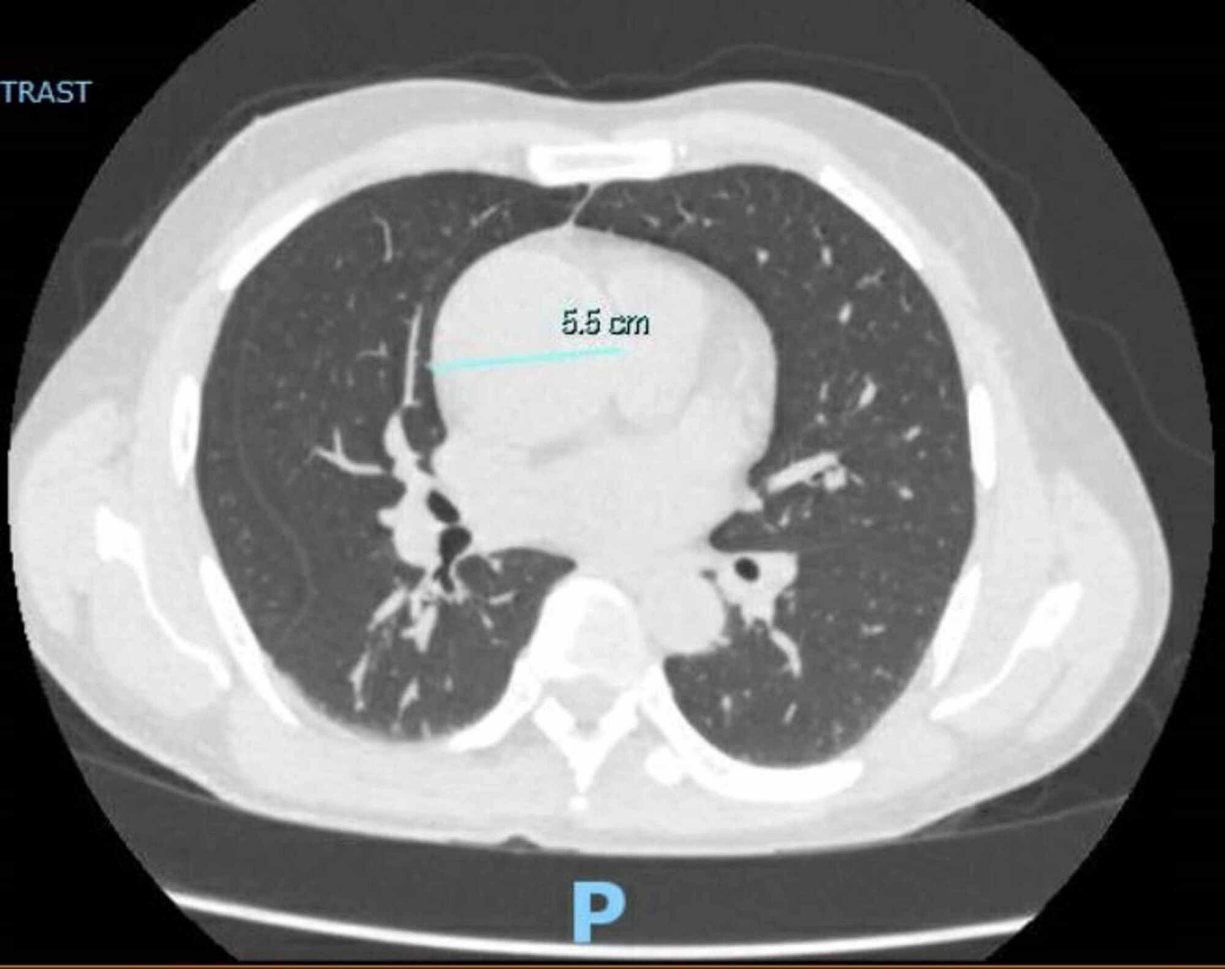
Computerized tomography (CT) angiogram of the chest showing ascending aortic aneurysm up to 5.5 x 5.2 cm in diameter without aortic dissection or intramural hematoma.
